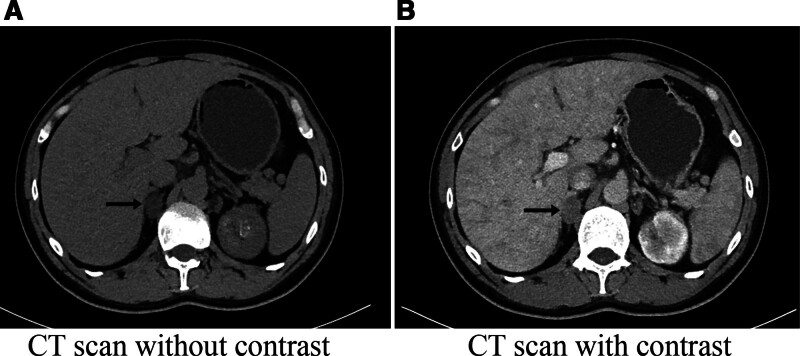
Abdominal computerized tomography (CT) image. The abdominal CT scan reveals a 23 mm × 16 mm nodule in the right adrenal gland. (A) The nodule appears as a relatively low-density area (arrows). (B) Mild enhancement is observed following contrast administration (arrows).
